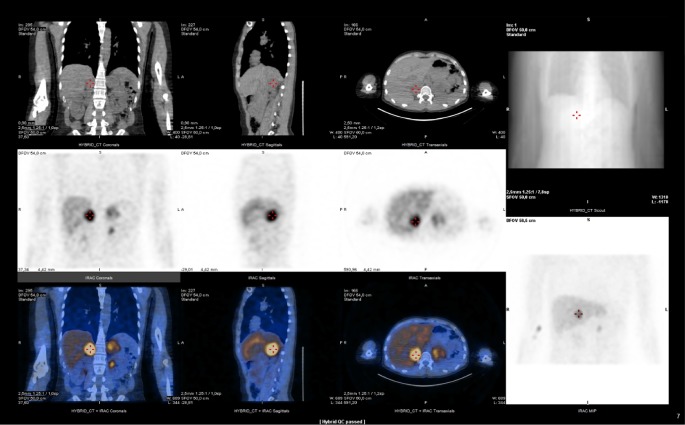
^123^I-MIBG SPECT/CT showing increased uptake of the tracer in both adrenal glands, with right predominance.
